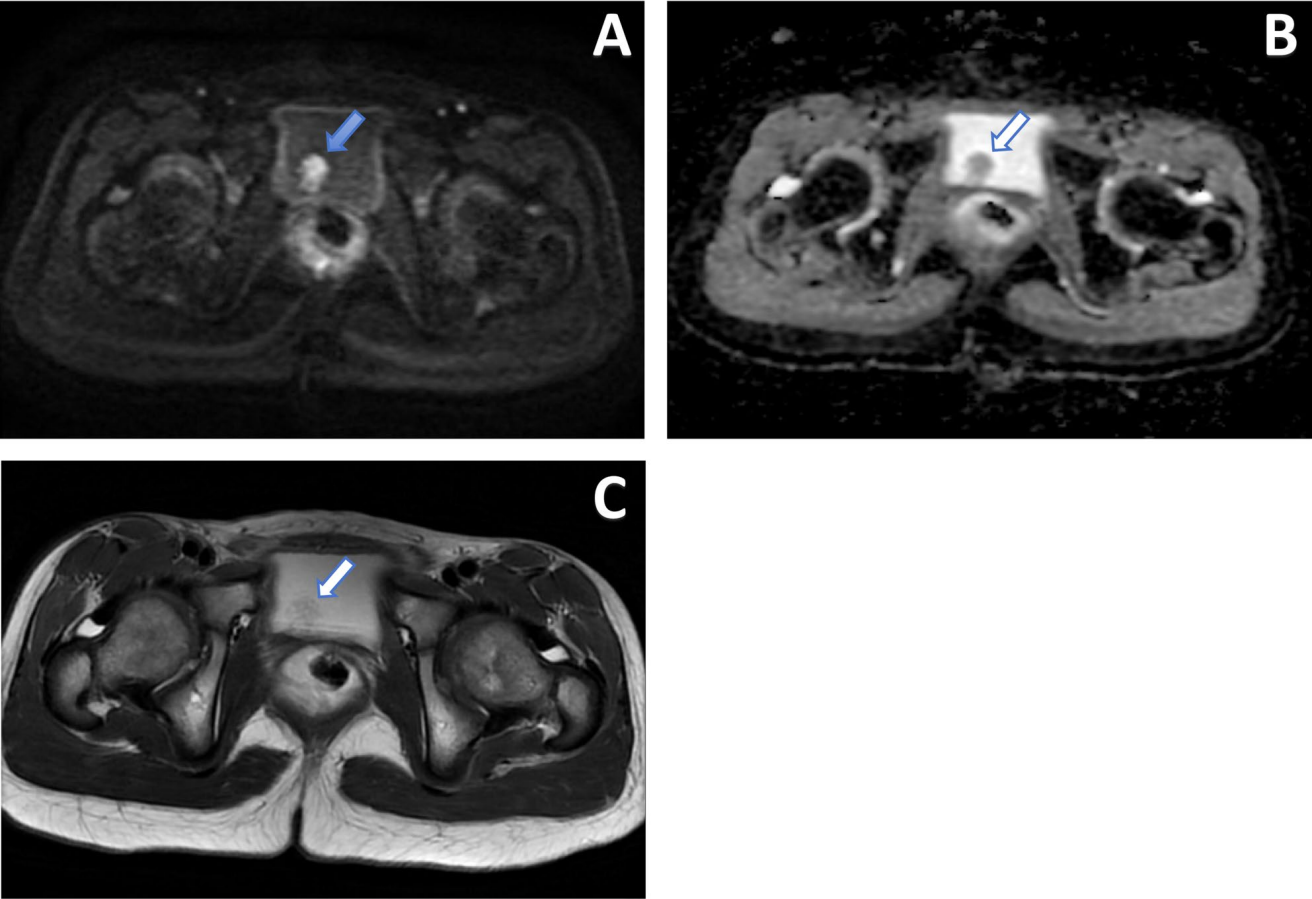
MRI findings of a bladder mass on the right lateral wall. A Axial diffusion-weighted image (DWI) shows high signal intensity. B Axial apparent diffusion coefficient (ADC) map shows corresponding low signal intensity. C Axial T2-weighted image (T2WI) shows an intermediate signal intensity mass (arrows)
